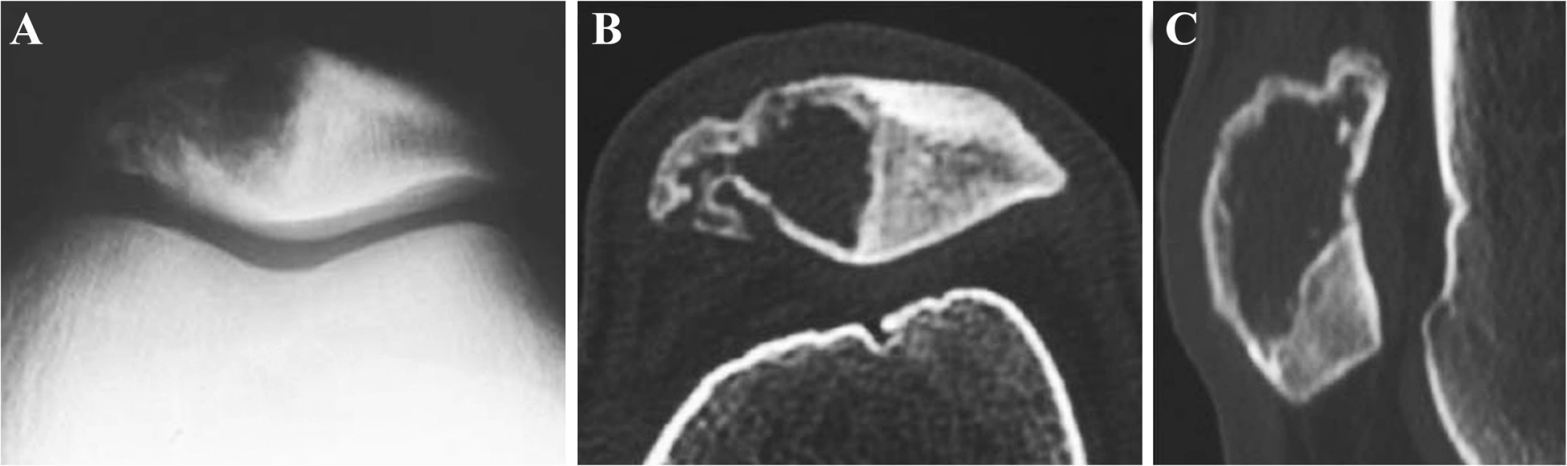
Osteosarcoma of the patella. (A) Plain radiograph and (B, C) CT images reveal a lytic lesion in the patella with partial cortical disruption.
